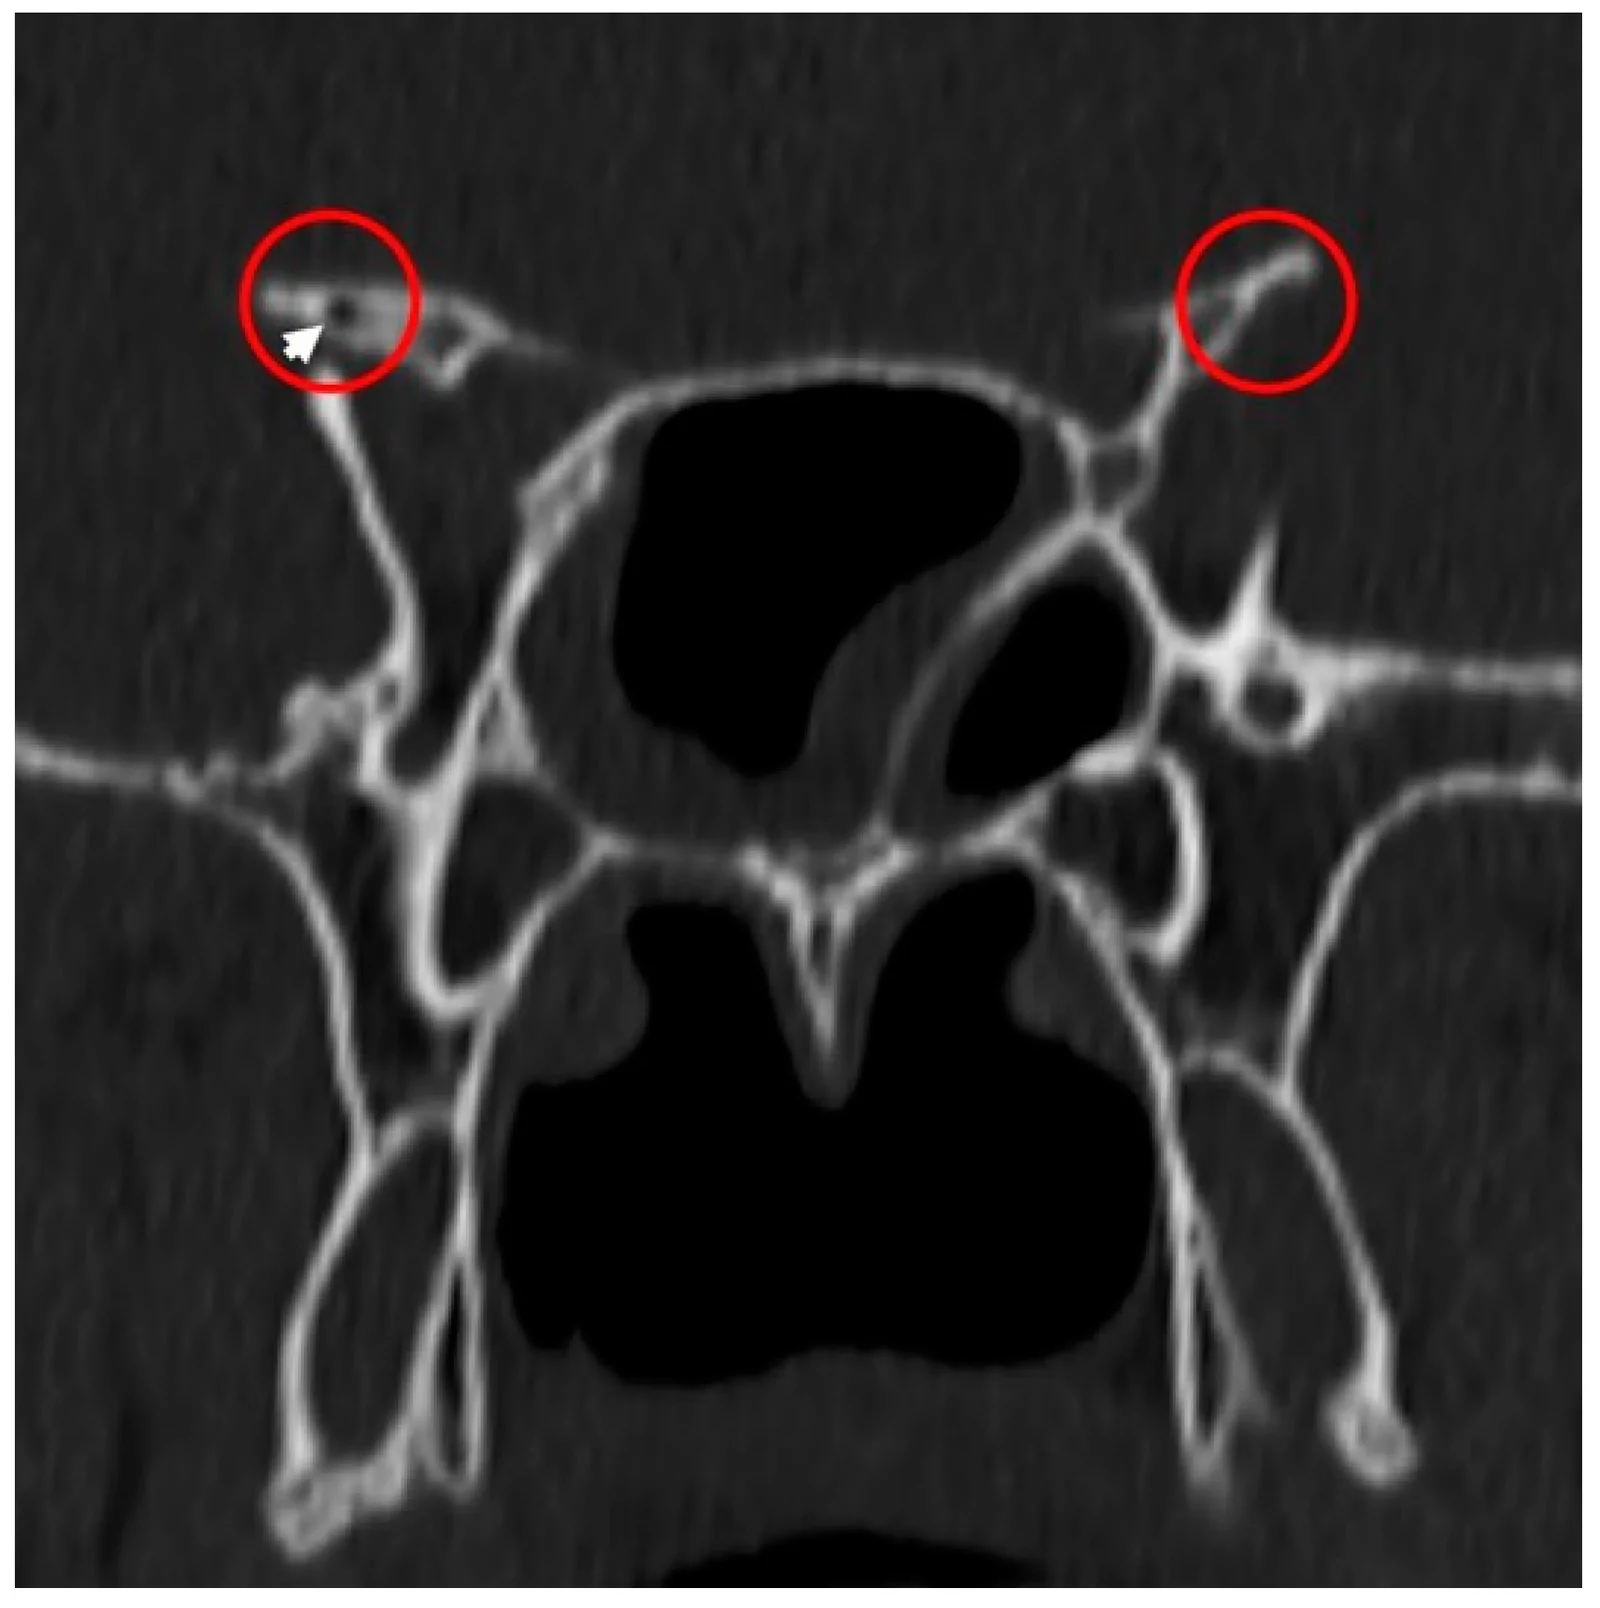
Representative coronal CT image demonstrating maxillary strut pneumatization. The white arrow indicates a visible air-containing cell within the maxillary strut. The red circles indicate the location of the maxillary struts.
